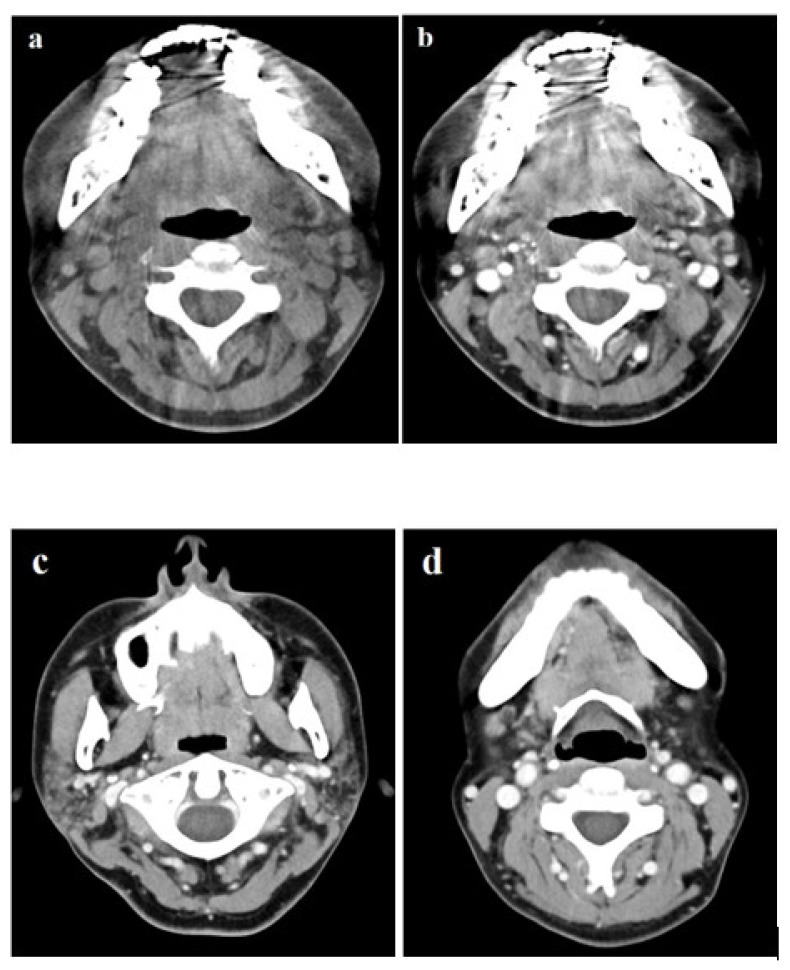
The lesions are not visible on non-enhanced axial (a) and enhanced axial CT (b) images owing to the presence of severe beam hardening artifacts by dental prosthesis. The contrast-enhanced CT (c) image shows multiple granulonodular opacities with partially fatty and mildly atrophic changes in both parotid glands. The contrast-enhanced CT (d) shows total fatty degeneration of both submandibular glands, however, glandular volume is conserved.
